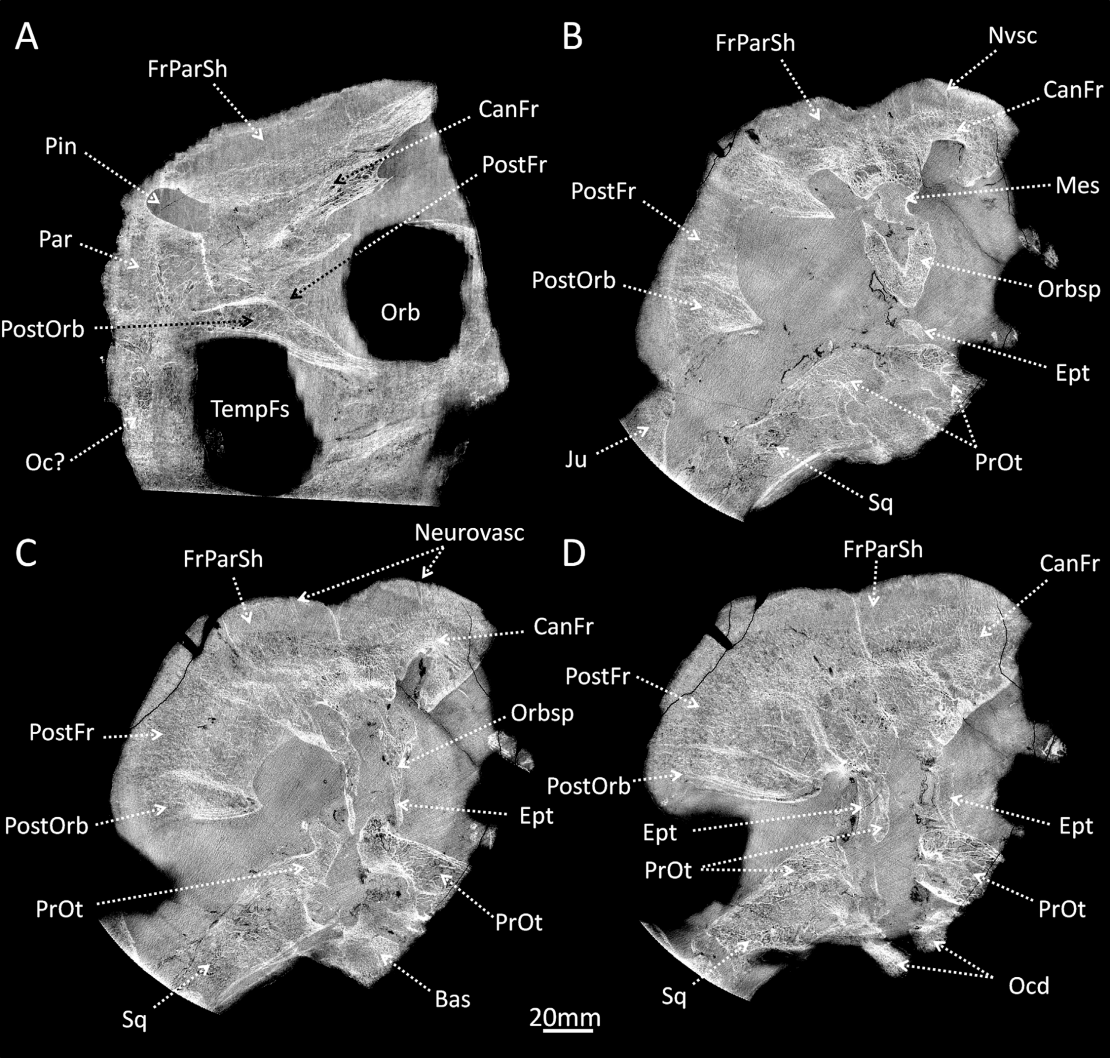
CT images of the braincase of *Moschops capensis* AM6556. A, longitudinal section. B-D, transverse sections. Abbreviations: Ept, epipterygoid; CanFr, cancellous frontal bone; FrParSh, frontoparietal shield; Ju, jugal; Mes, mesethmoid; Nvsc, neurovascular canal; Oc?, unidentified occipital bone; Ocd, occipital condyle; Orb, orbite; Orbsp, orbitosphenoid; Par, Parietal; Pin, pineal tube; PostFr, postfrontal; PostOrb, postorbital; PrOt, prootic; Sq, squamosal; TempFs, temporal fenestra. Scale bar: 20mm.
